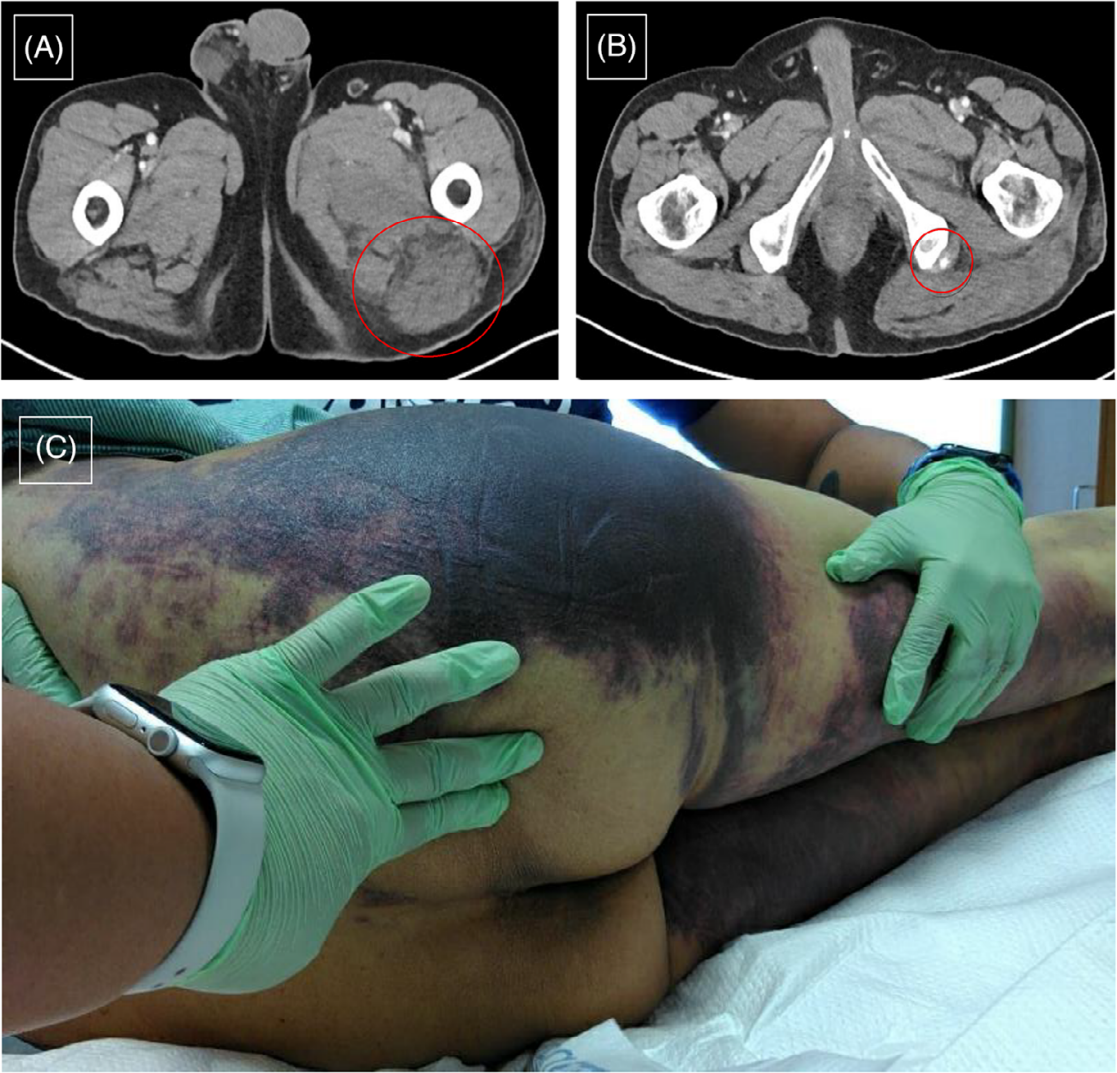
(A) Computed tomography (CT) abdomen/ pelvis axial section showing diffuse intramuscular hematoma formation throughout the posterior compartment of the left upper thigh. (B) CT axial section demonstrating active bleeding, likely from the left inferior gluteal vessels. (C) Physical examination showed extensive ecchymoses from retroperitoneal hematoma, tracking down the flank into the leg.
